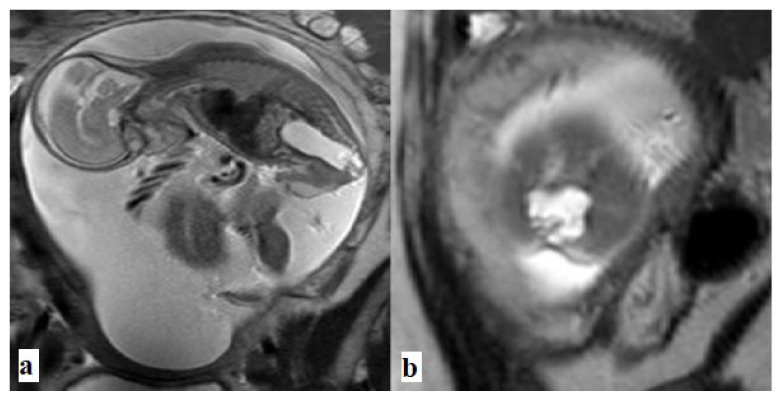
Type IV sacrococcygeal teratoma. a. Sagittal, and b. Axial T2-weighted images show tumor developed entirely in the fetal pelvis. The urinary bladder is displaced and vertical dimension is increased and contours are lobulated with increased thickness. There is also polyhydramnios.
